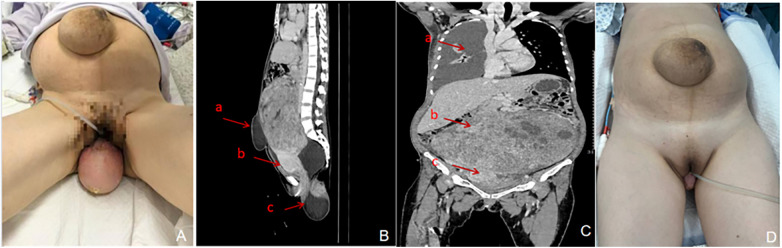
Presentation and imaging findings at admission, and changes after preoperative decompression. (A) Supine clinical photograph of the patient on the day of admission, showing marked abdominal distension, a giant umbilical hernia, and posterior vaginal wall prolapse. (B) Sagittal reconstruction of an abdominal CT scan obtained at admission. Arrow a indicates the giant umbilical hernia (measuring approximately 121 × 62 × 117 mm); arrow b points to the uterus; arrow c indicates the posterior vaginal wall prolapse (measuring approximately 95 × 90 × 98 mm). (C) Coronal reconstruction of a chest CT scan obtained at admission. Arrow a indicates right-sided massive pleural effusion with compressive atelectasis; arrow b points to the huge pelvic mass (later pathologically confirmed as a uterine leiomyoma, measuring approximately 275 × 191 × 231 mm); arrow c marks the normally positioned uterine body. (D) Clinical photograph of the patient on the day of surgery (hospital day 25), after completion of the preoperative preparation phase. Compared with panel A, marked improvement in abdominal distension, umbilical hernia, and vaginal prolapse is evident following aggressive drainage and supportive therapy.
